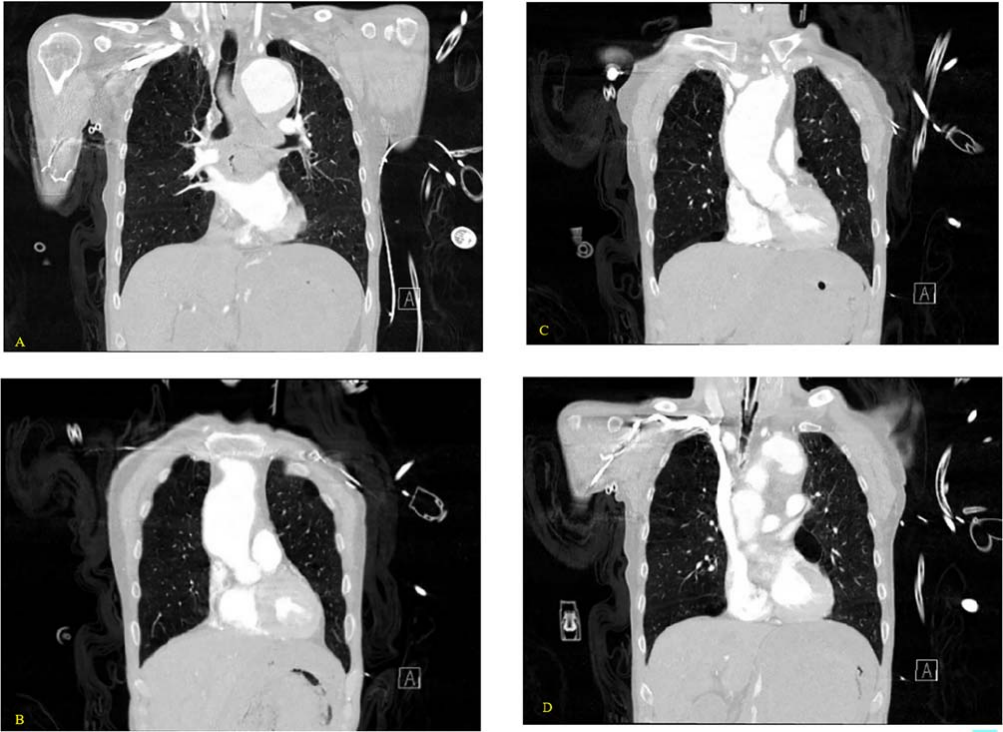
(A–D) Coronal CT chest with contrast reveal the descending aortic aneurysm of at least 6.5-cm diameter. Deep ulceration in the descending aorta at approximately the level of the pulmonary artery bifurcation. The ulceration has eroded into the esophagus. Extravasation of contrast is observed from the ulceration into the esophagus.
